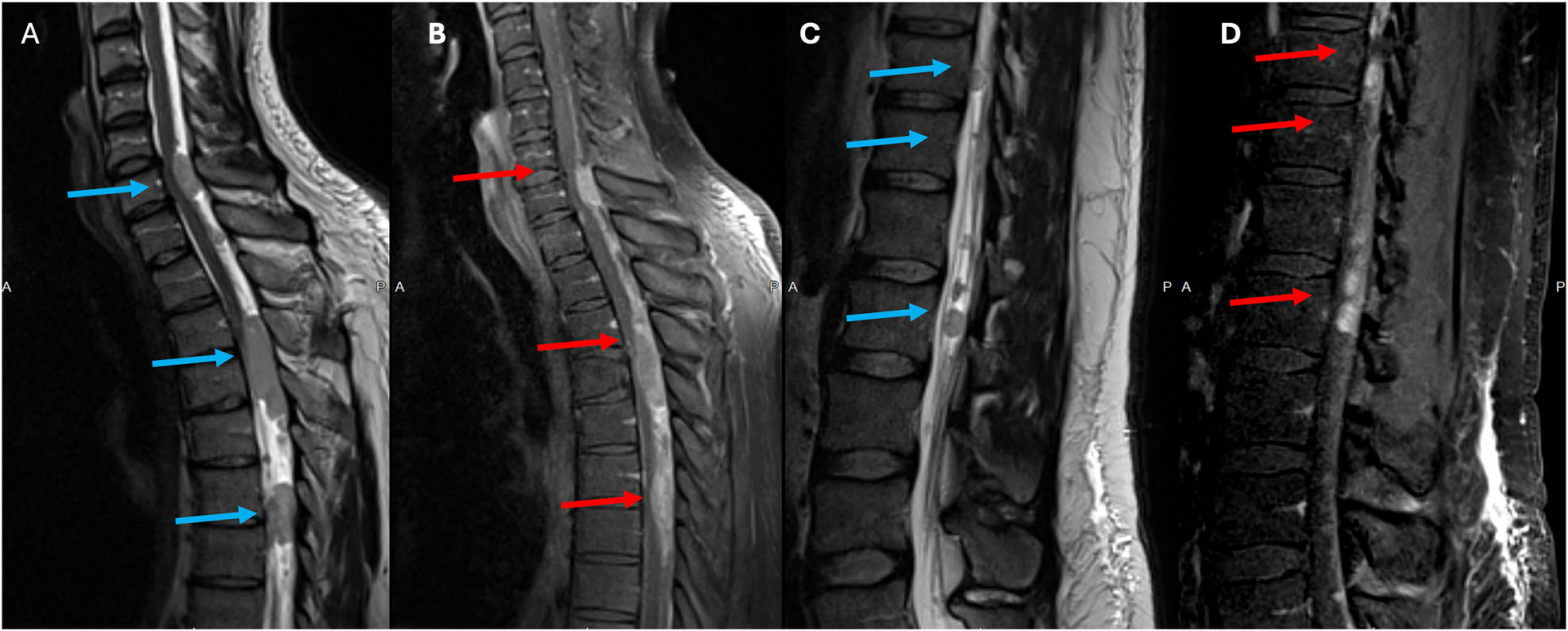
Fifty-four-year-old male patient with headaches and gait disturbances. Whole spine MRI with T2 (A, C) T1 postcontrast images (B, D) showing T2 well-defined, confluent, T2 isointense (blue arrows) and homogeneously enhancing intradural lesions (red arrows) starting from C7 and extending caudally towards L1. The lesions are displacing anteriorly and compressing the spinal cord
